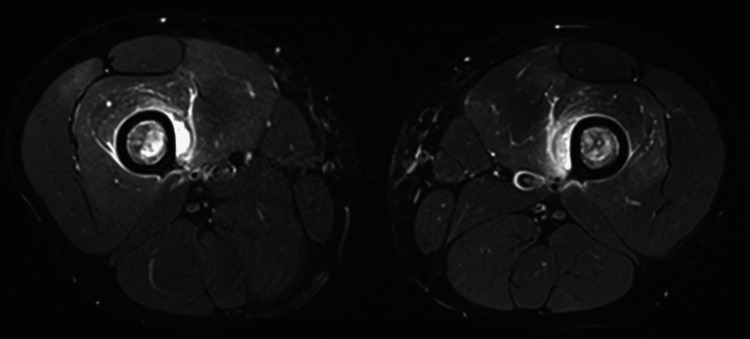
Day 10 axial MRI scan An axial T1 turbo spin echo (TSE) MRI image was taken of the lower limbs on Day 10 after hospital admission. The image showed a significant reduction in muscle edema compared with the Day 6 image, however, the periosteal lifting and bone infarctions remained essentially unchanged.
